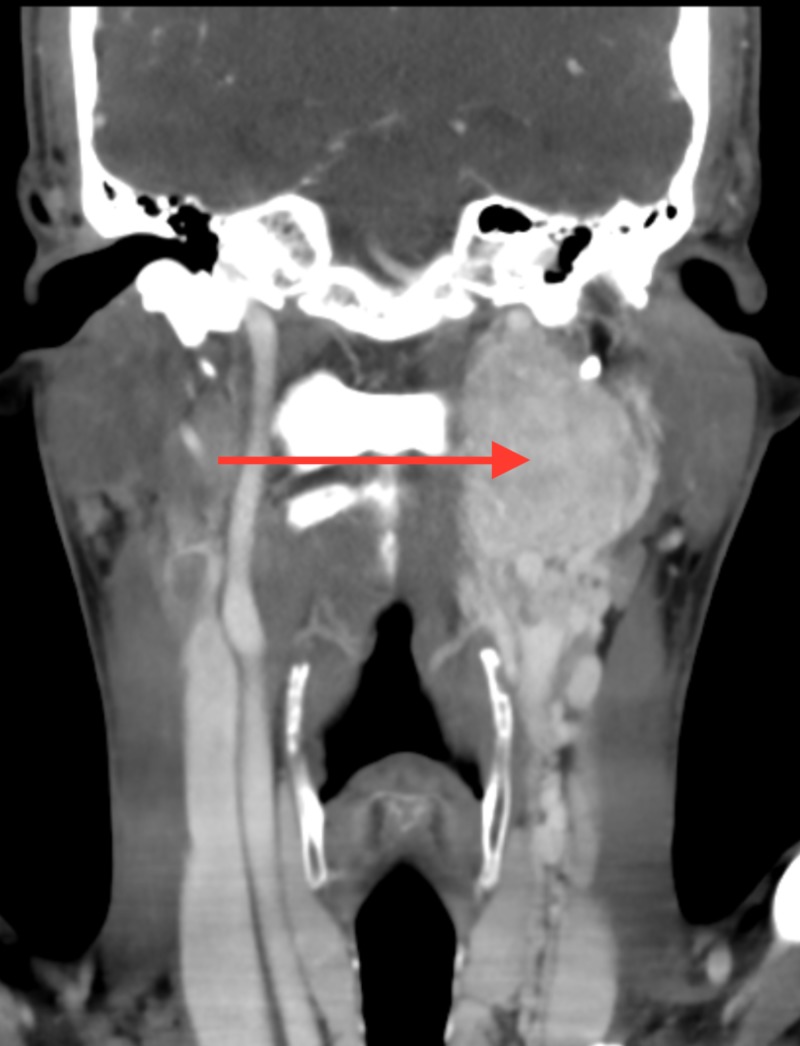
CT neck with IV contrast (coronal view). Large avidly enhancing mass within left carotid sheath extending from level of hyoid to jugular foramen at skull base.
